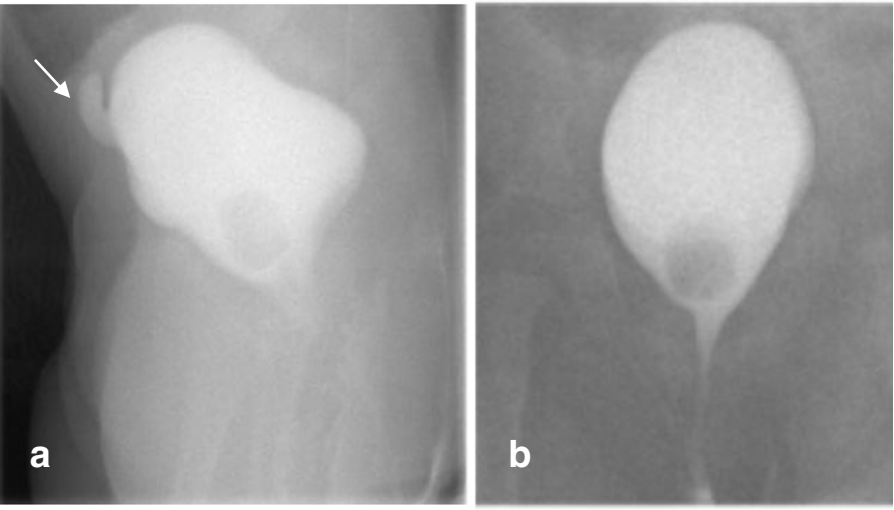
a Lateral and (b) AP projections of MCUG: Contrast opacified a pear-shaped structure in the pelvis at the location of the urinary bladder, with a tubular outpouching from its superior aspect (arrow). The contrast filled structure was thought to represent a urinary bladder with a diverticulum or reflux into an ectopic ureter. After assessment with MRI this structure was the vagina, and the outpouching was an ectopic ureter draining to the anterior wall of the vagina
